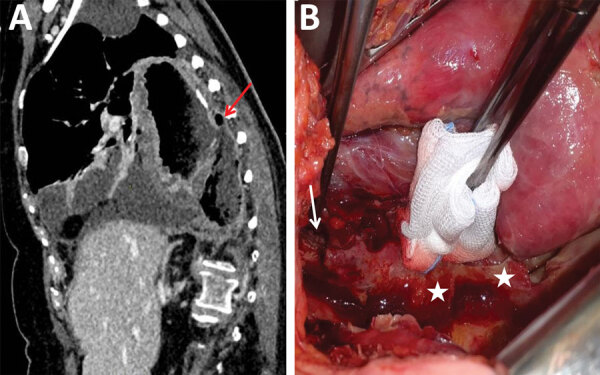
Clinical and radiologic characteristics of mediastinitis caused by *Kazachstania bovina* (patient 2), Strasbourg, France. A) Computed tomography image demonstrating stomach ulceration (arrow), mediastinitis, and pleuritis. B) Photograph taken after right-side thoracotomy, showing posterior stomach ulceration (arrow) and false membranes (stars). Culture of biopsy samples grew *K. bovina*, *Candida albicans*, *C. glabrata*, and bacteria.
